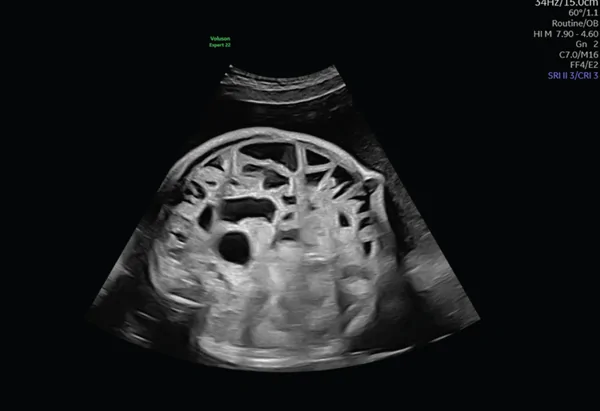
Case 1, dilated bowel loops at 35 weeks of gestation with “honeycomb aspect” and polyhydramnios in patient with a SLC9A3 mutation (ultrasound image).
